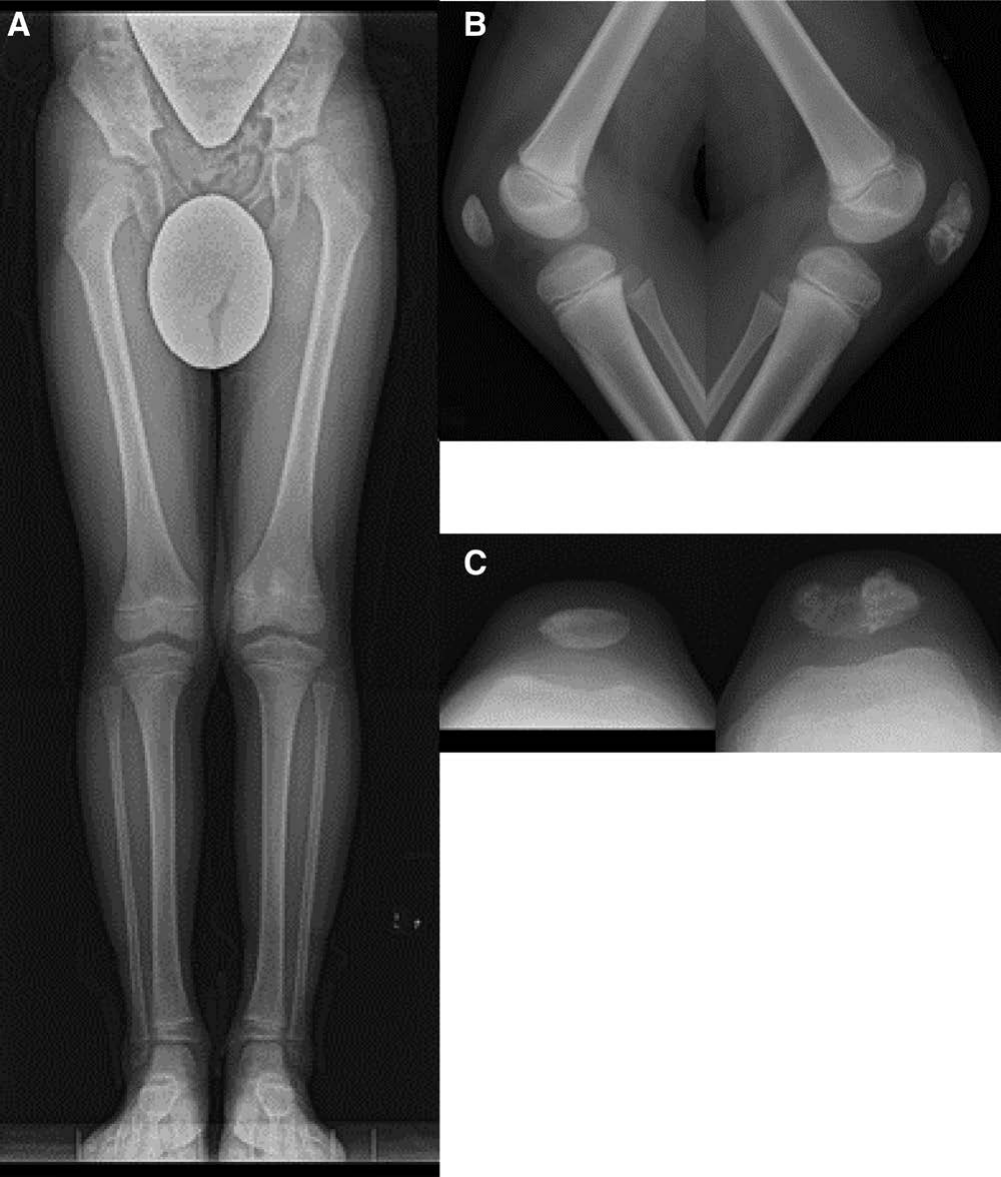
Radiographs obtained 3 years after the onset. (A) A full-length standing anteroposterior radiograph of the lower extremity. Lateral (B) and sky-line (C) views of both knees.
